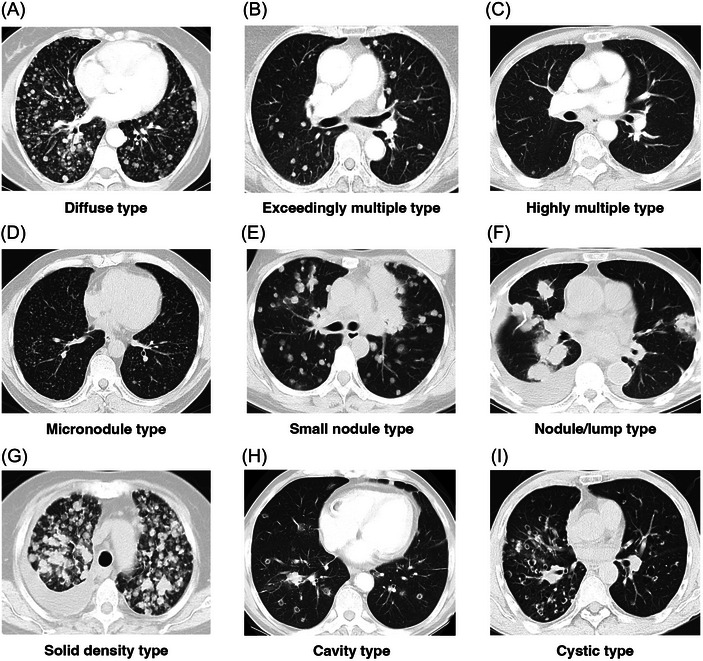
CT imaging of multiple pulmonary hematogenous metastases and subtype of MPHM. (A) Diffuse type: nodules are diffusely distributed in lung. (B) Exceedingly multiple type: more than 10 nodules which are not diffusely distributed. (C) Highly multiple type: more than three but less than or equal to 10 nodules. (D) Micronodule type: 0< maximum diameter ≤5 mm. (E) Small nodule type: 5 mm< maximum diameter ≤10 mm. (F) Nodule/lump type: 10 mm< maximum diameter. (G) Solid density type: the components of the nodules are all solid. (H) Cavity type: the centre of the nodule has formed a cavity. (I) Cystic type: tumour grow along the cyst cavity of original emphysema. MPHM, multiple pulmonary hematogenous metastases.
